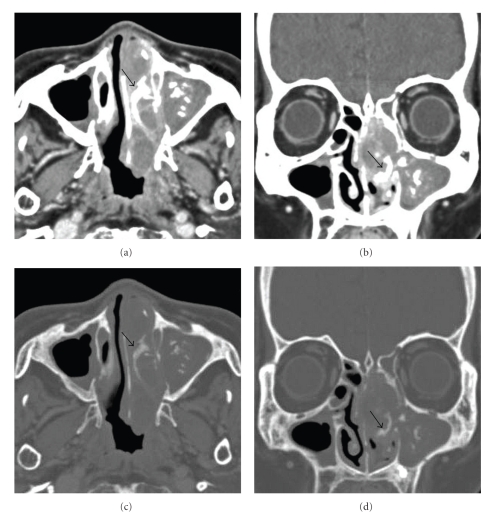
Horizontal (a) and axial (b) enhanced computed tomography (CT) showed an inhomogeneously enhancing mass that filled the left nasal cavity. A low-density lesion with metal-dense spots in the left maxillary sinus, indicating mycetoma, was also observed. Bone window images ((c), (d)) also showed a new bone formation in the tumor. Black arrows indicate a new bone formation.
